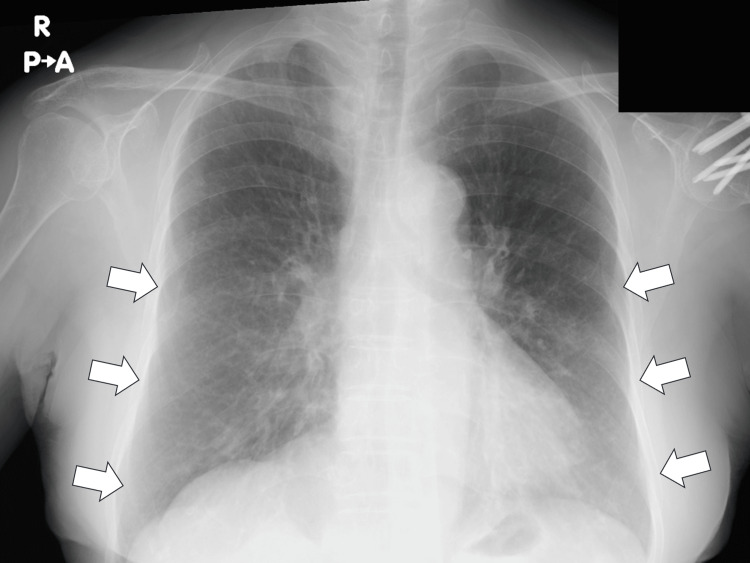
Chest X-ray demonstrating patchy ground-glass opacities in the bilateral middle lung fields (white arrows)
